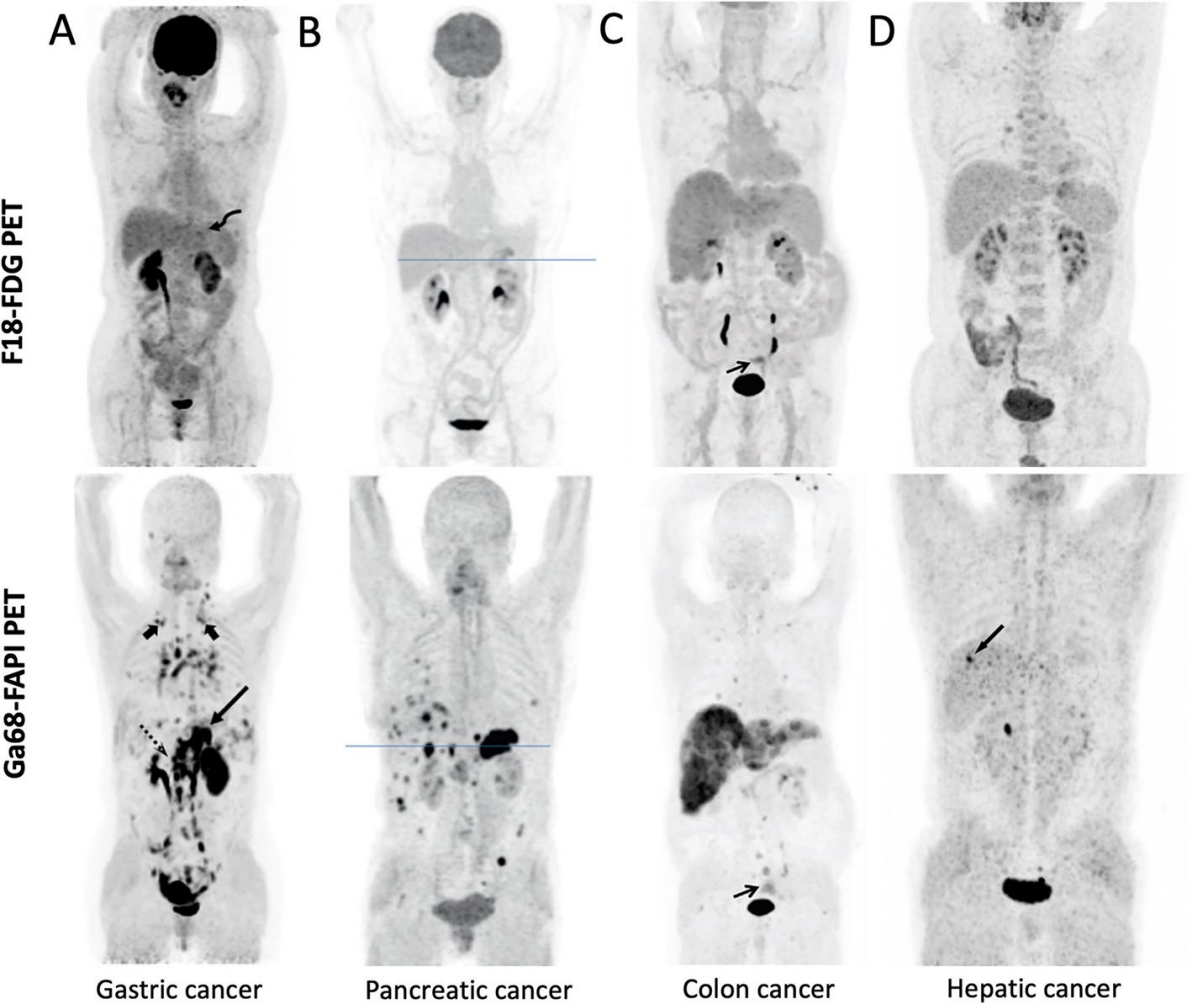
Superiority of FAPI PET over FDG PET in Gastrointestinal cancers. A Patient with poorly differentiated gastric adenocarcinoma underwent FAPI PET and FDG PET imaging. FAPI PET revealed high uptake in gastric cardia (slender arrow), paraaortic lymph nodes (dashed arrow) and supraclavicular lymph nodes (short arrows) which were negative on FDG PET. FDG PET revealed increased uptake in gastric anastomosis (bent arrow) which was negative on FAPI PET and eventually was confirmed as residual gastritis by biopsy. B Patient with pancreatic cancer underwent FAPI and FDG PET imaging. FAPI outperformed FDG PET in detecting primary tumours and metastatic lesions such as perihepatic lesions and pulmonary lesion which was confirmed as PDAC metastasis. C Patient with colon cancer underwent FAPI and FDG imaging for an initial assessment. The uptake in the primary tumour (black arrows) and metastatic lesions was higher on FAPI PET than that of FDG PET. D In a patient with moderately differentiated hepatocellular carcinoma, FAPI PET revealed a strongly FAPI-avid lesion in the right hepatic lobe with no positive findings on FDG PET. (Figures adapted from A Zhang et al. [43], C Pang et al. [42] and D Wang et al. [49], under a CC BY license. B was originally published in *JNM*. Röhrich M, Naumann P, Giesel FL, Choyke PL, Staudinger F, Wefers A, Liew DP, Kratochwil C, Rathke H, Liermann J, Herfarth K, Jäger D, Debus J, Haberkorn U, Lang M, Koerber SA. Impact of 68Ga-FAPI PET/CT Imaging on the Therapeutic Management of Primary and Recurrent Pancreatic Ductal Adenocarcinomas. J Nucl Med. 2021;62(6):779–786. © SNMMI [50])
